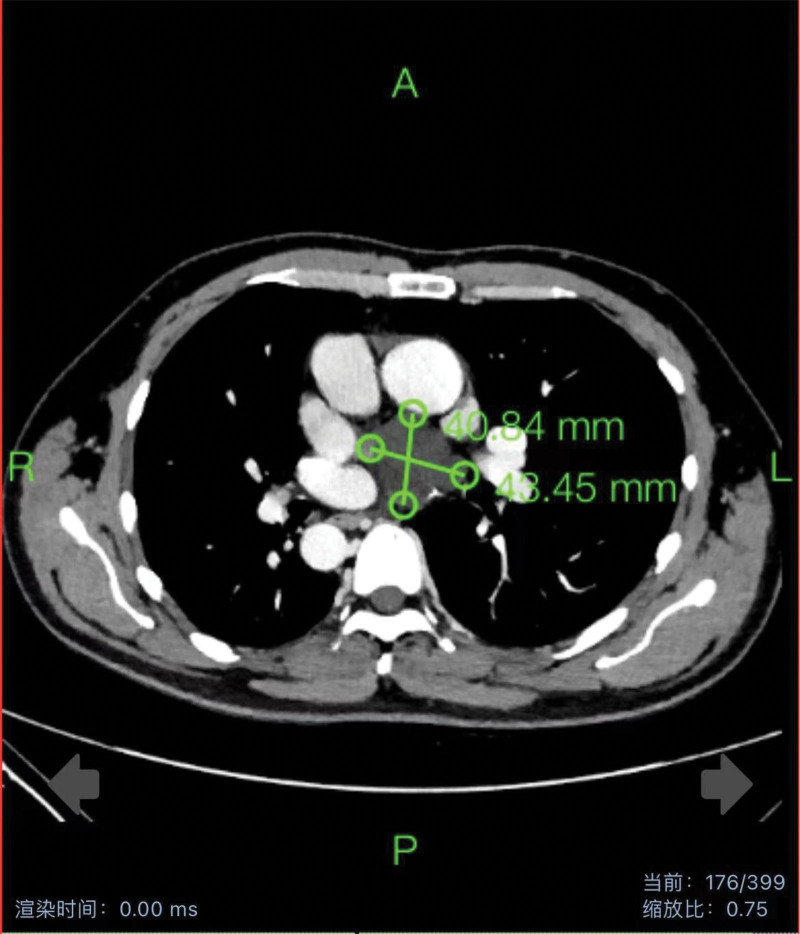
Chest contrast-enhanced CT scan findings: Post-contrast CT scan of the chest reveals a cystic lesion below the bifurcation of the mediastinal trachea, measuring approximately 41*43mm in size.
